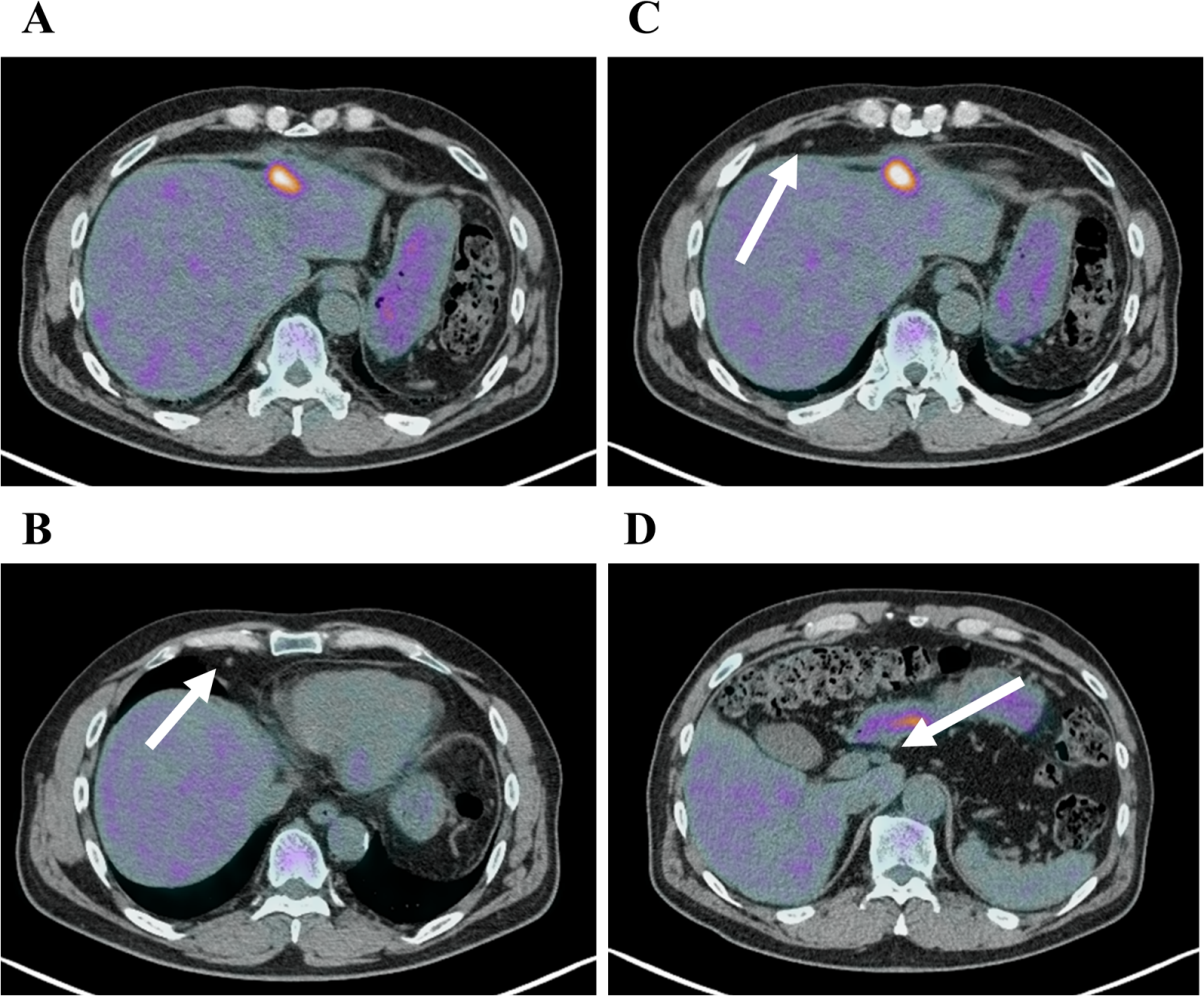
Abdominal fluorodeoxyglucose/positron emission tomography after chemotherapy and transcatheter hepatic arterial injection. A Accumulation of fluorodeoxyglucose (FDG) is observed in the primary tumor with reduced size after the treatment with systemic chemotherapy and transcatheter hepatic arterial injection. B–D Accumulation of FDG is not observed in the perihilar lymph node and multiple lymph nodes around the heart. The size of the lymph nodes is reduced after the treatment (white arrows)
